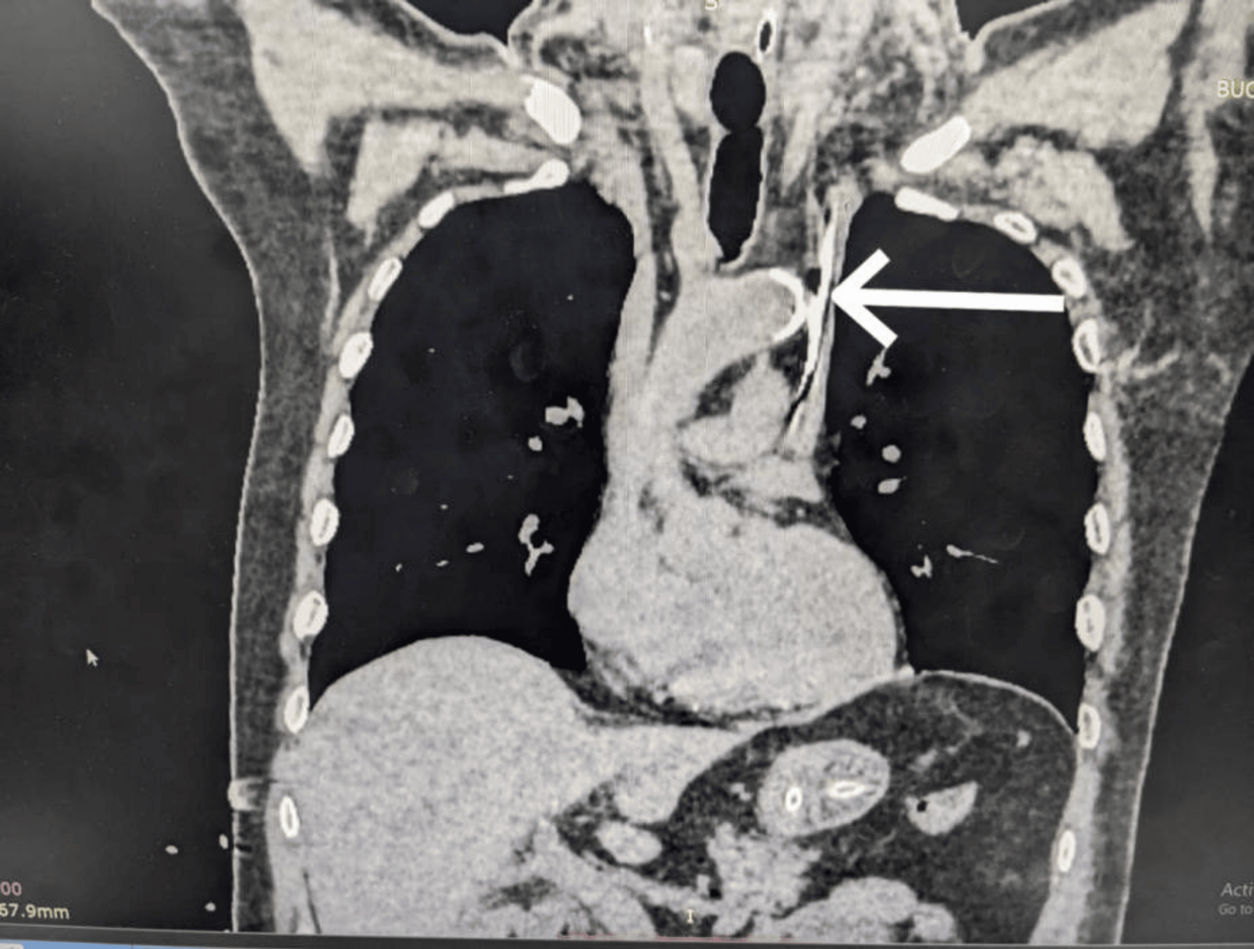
CT chest with the coronal view showing bilateral superior vena cava (SVC)
